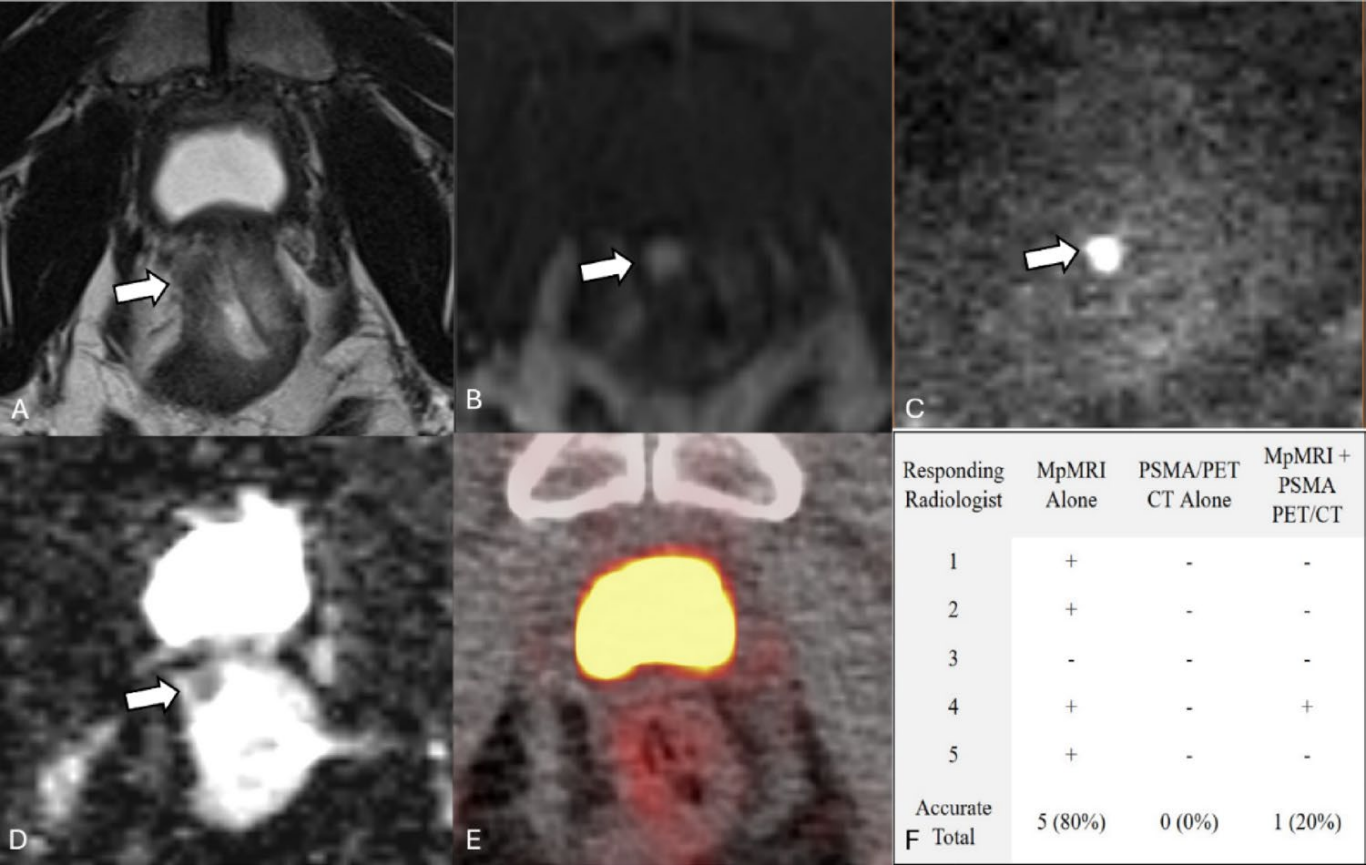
57-year-old patient with a right anterior rectum lesion with early enhancement and restricted diffusion on mpMRI but no focal uptake on PSMA PET/CT. This case depicts a 57-year-old status-post prostatectomy indicated for a primary adenocarcinoma with a Gleason score of 5 + 4 = 9 with a T3b pathological stage. His PSA was measured to be 0.4 ng/mL at the time of imaging. MpMRI (A T2, B Arterial enhancement T1, C DWI, D ADC) shows a 5–10 mm T2 dark lesion with early enhancement and restricted diffusion in the right anterior rectum (white arrow). 18 F-piflufolastat PET/CT (E) shows no focal uptake above background in this region. MpMRI and PSMA PET/CT were performed 23 days apart. MpMRI alone yielded a higher diagnostic accuracy than the combination of mpMRI and PSMA PET/CT (F)
